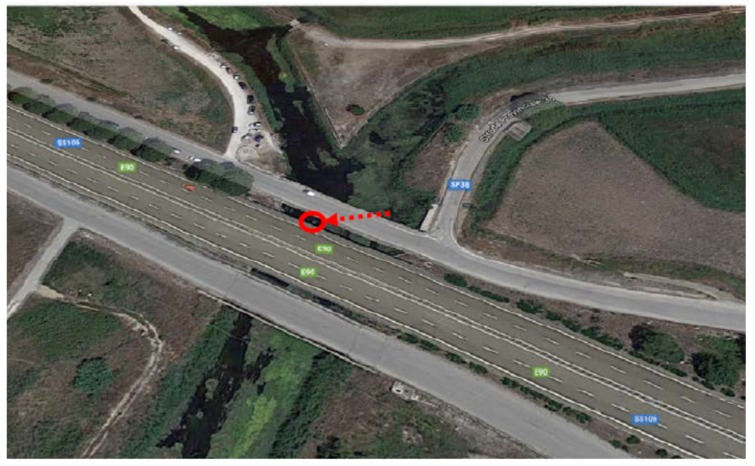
Location of the monitoring station on the road bridge, with a camera and ultrasound meter.
